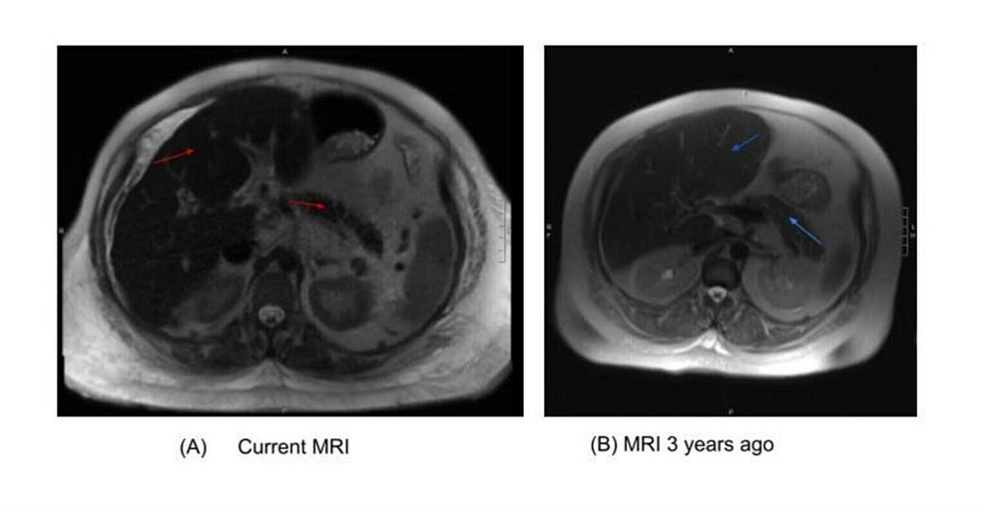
Comparison of Contrast-enhanced MRI-Axial HASTE section: Iron deposition depicted by dark black color in liver and pancreas - red arrows in image A vs. normal liver and pancreas 3 years ago depicted by grey color in liver and pancreas - blue arrows in image B.
